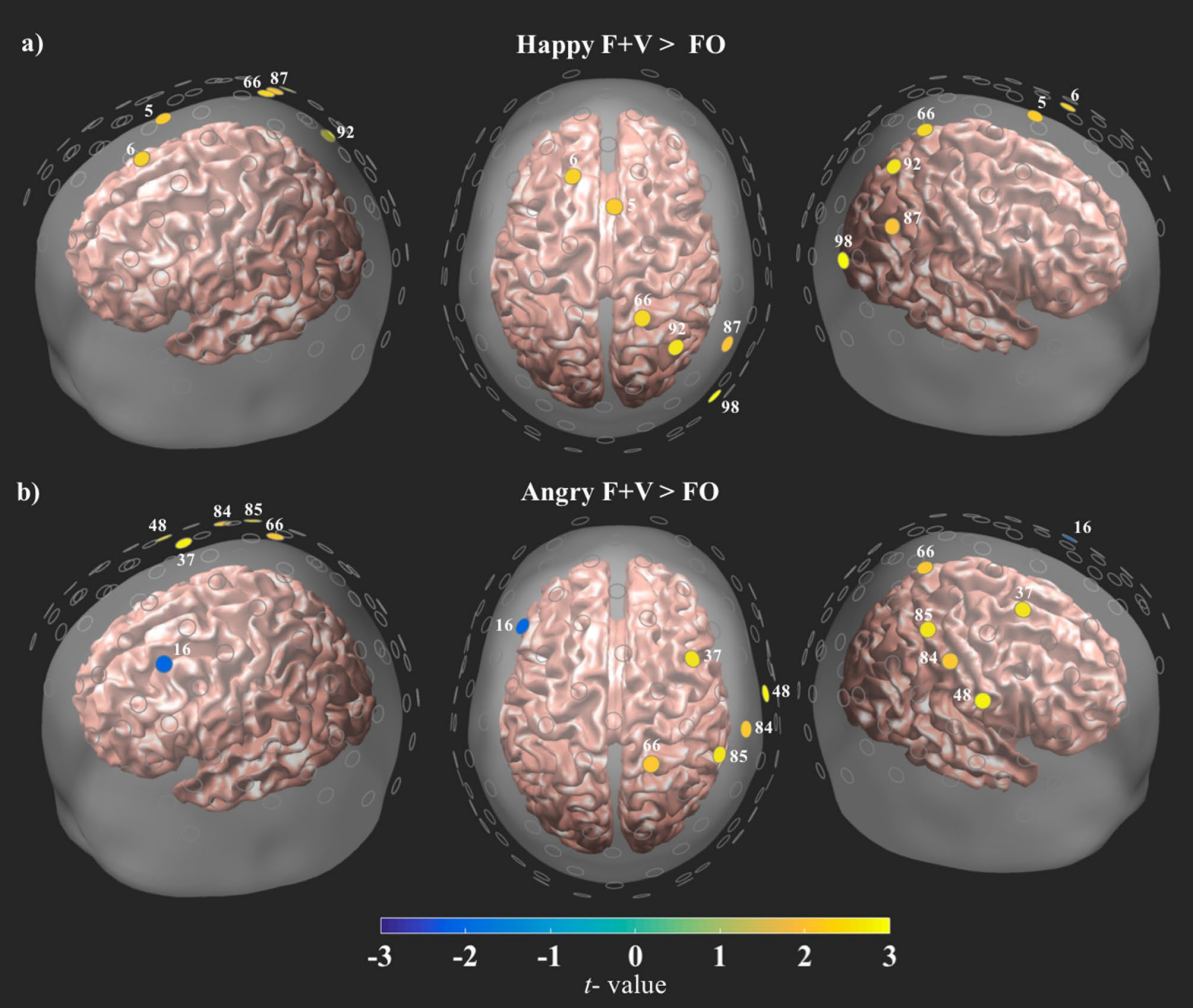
Results of all the bimodal F + V condition contrasts. Channel locations in Fig. 1 and all other results figures are shown overlaid onto a semi-transparent scalp and standard single subject MRI template from SPM 12 for ease of spatial reference. All channels are plotted as gray circles. Channels with significant differences in HbO are shown in yellow or blue, with the associated channel number shown in white. The magnitude and direction of t-values is represented by the color bar shown on the bottom of the figure. All channels survived FDR correction at $$\hbox {q} = .05$$.
